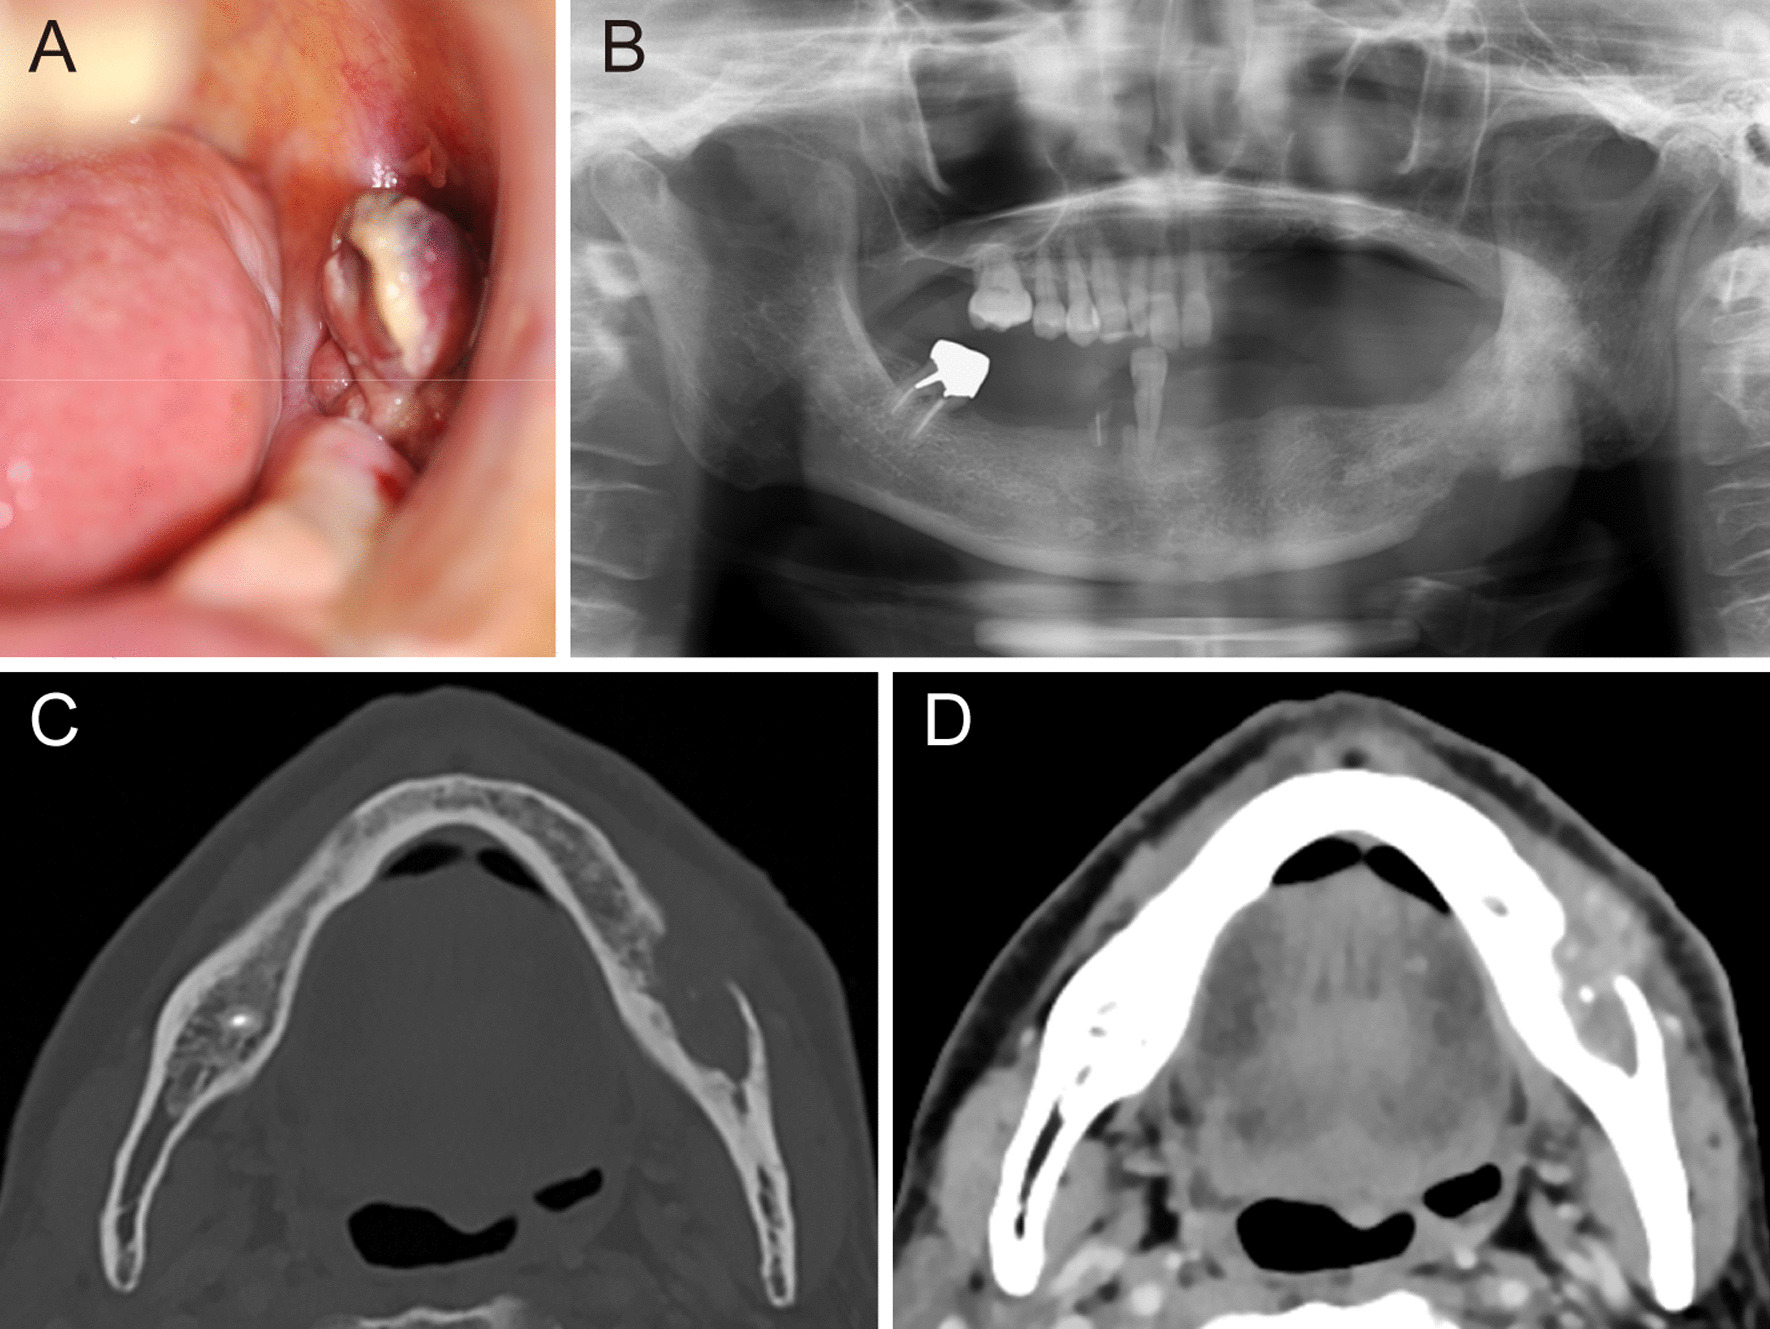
Clinical and radiological findings of the polypoid mass arising in the left molar region of the mandible 15 years later. A Intraoral view showing a polypoid mass (10 mm in diameter) with an ulcerated surface in the left lower molar region of the lower gingiva. B Panoramic radiography showed a moth-eaten bone resorption pattern. C Computed tomography (CT) image, bone window, axial section, and D contrast-enhanced CT image, soft-tissue window, axial section revealed an enhancing mass lesion with irregular resorption of the mandible
